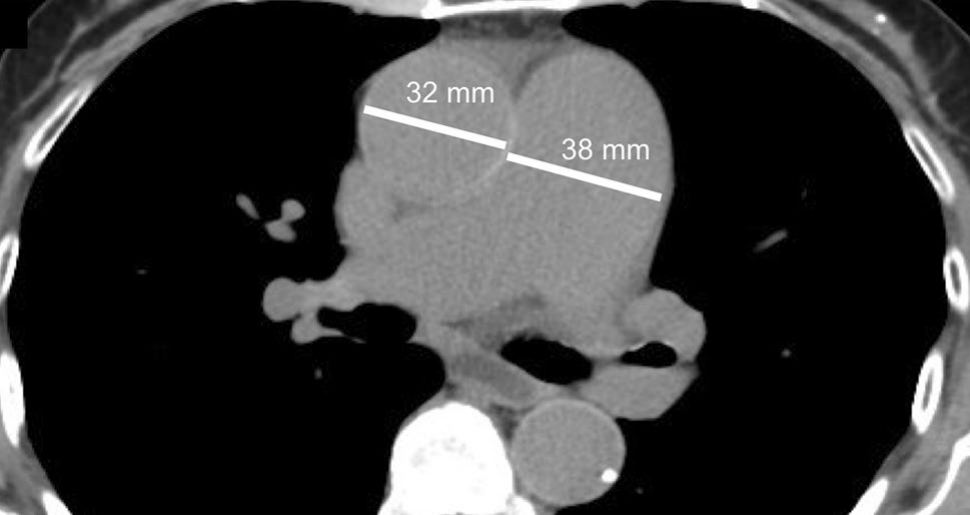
Pulmonary hypertension: the main pulmonary arterial diameter measured at the level of its bifurcation on the axial CT scan is 38 mm and the ratio of the diameter of the main pulmonary artery to the diameter of the ascending aorta is greater than 1:1
